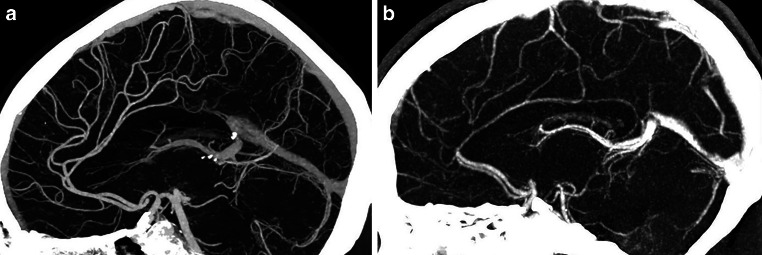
Two exemplary patients with the same clinical characteristics. a Maximum intensity projection with a slice thickness of 17.5 mm calculated on an UHR-CTA data set in sagittal view. b Maximum intensity projection with a slice thickness of 17.5 mm calculated on an NR-CTA data set in sagittal view. In contrast to NR-CTA, the significantly improved visualization of the small vessel branches in UHR-CTA can be considerably emphasized
